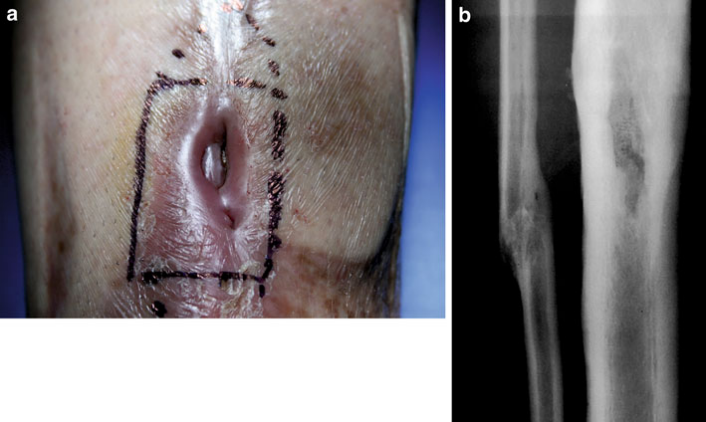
a The open fistula and the corresponding bone defect underneath b X-ray)
